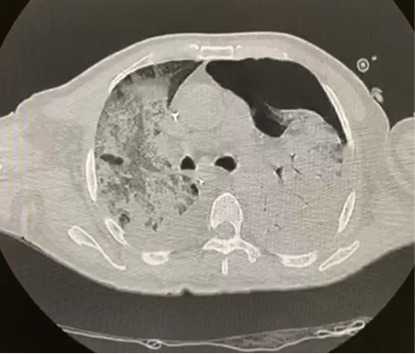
Chest CT scan showing bilateral air bronchogram in the lungs.
